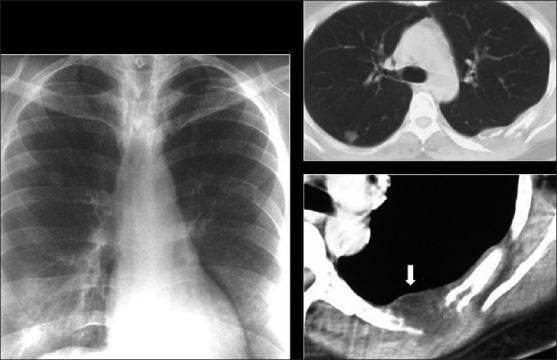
Cryptococcosis. Chest X-ray and computed tomography show lessfrequent manifestations of lung nodules and a chest wall abscess (arrow)
